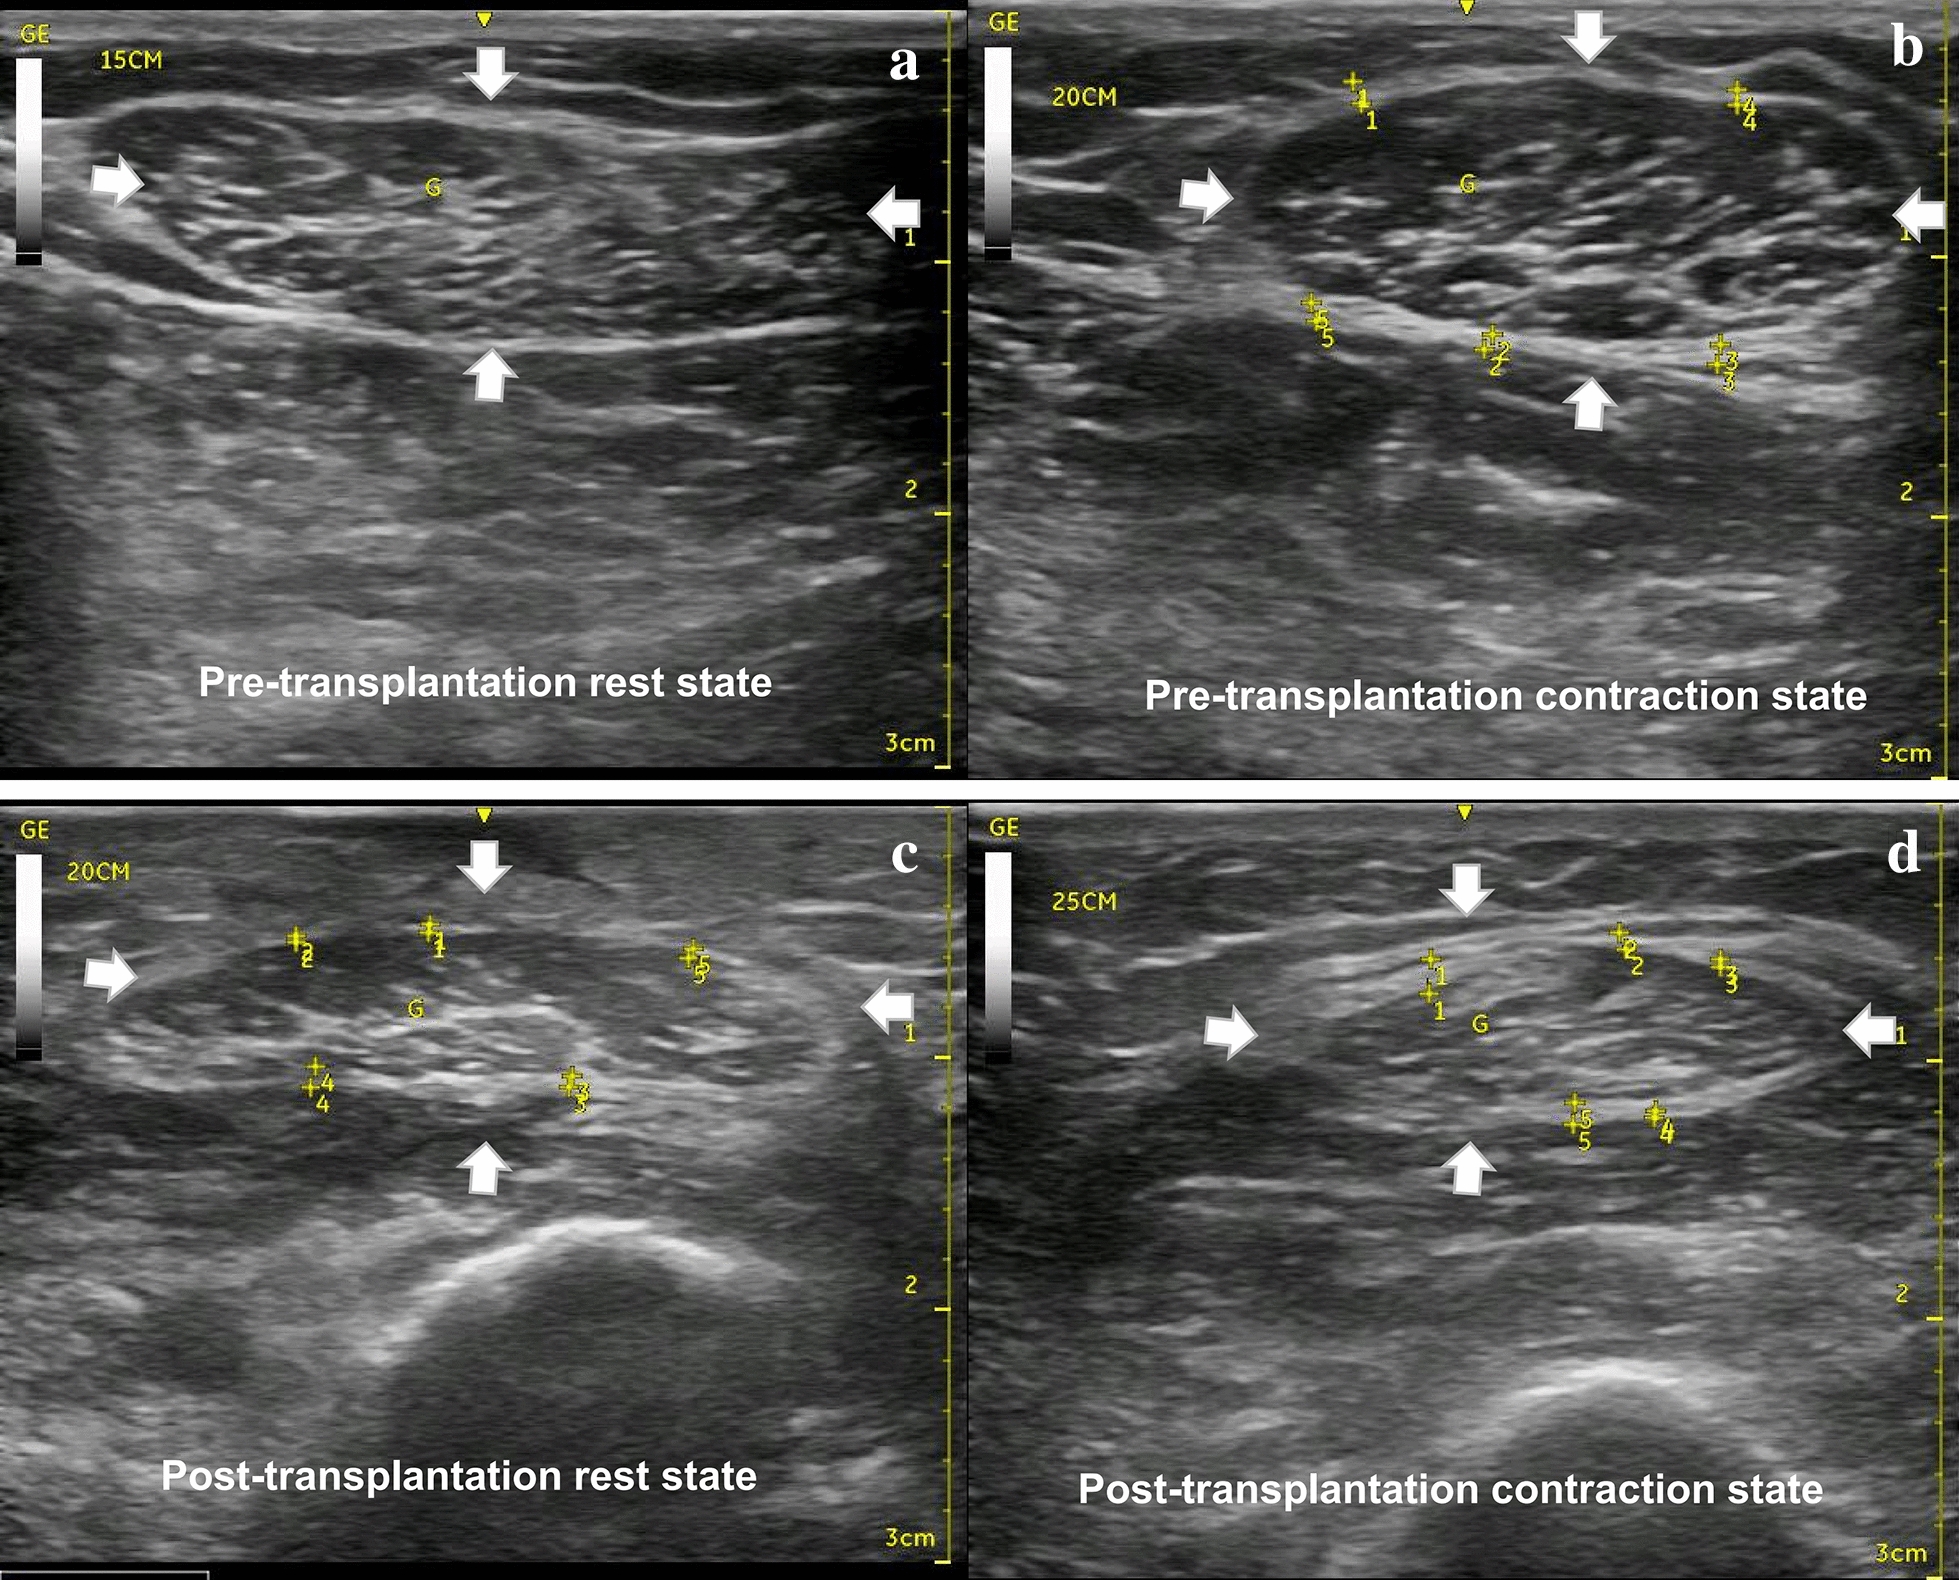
Ultrasound image of cross-sectional area of the gracilis muscle. The white arrows indicate muscle cross-section area (CSA). a Pre-transplantation rest state; b Pre-transplantation contraction state; c post-transplantation rest state; d post-transplantation contraction state. The ratio of pre- to post-transplant CSA value of gracilis muscle at rest state was defined as muscle bulk ratio (MBR). The ratio of CSA value at the contraction state to that at rest state was defined as contraction ratio (CR). The CR values of gracilis muscle before and after transplantation were defined as CR-2 and CR-1, respectively. MBR = CSA in c/CSA in a; CR1 = CSA in d/CSA in c; CR2 = CSA in b/CSA in a
